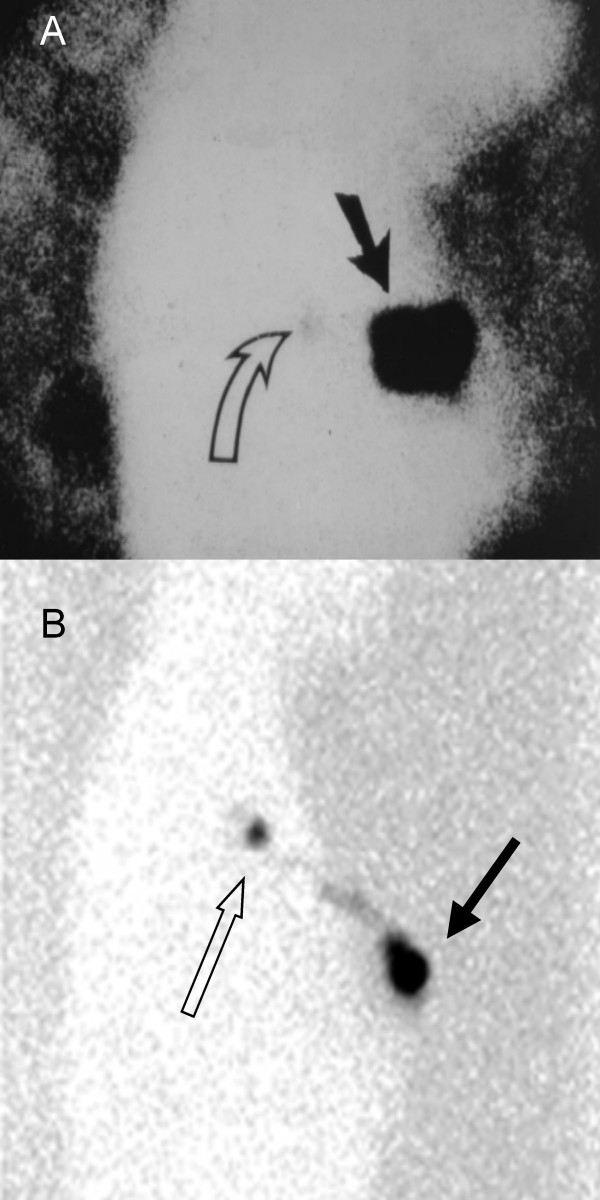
(A*) TOP: Labeled as a "typical" lymphoscintigraphy finding in an article disputing the value of lymphoscintigraphy, the lateral view depicts the poor quality of injection and imaging technique [37]. The injection site is represented by the solid arrow, the faint, barely visible SN by the open arrow. (B) BOTTOM: Right lateral view of typical/average result from optimized injection and imaging protocol showing injection sites (solid arrow) and bright sentinel node (open arrow) as well as lymphatic channel leading to sentinel node [38]. In many cases, even much brighter nodes than depicted in B are found. Reprinted from Am J Surg. 177, Burak WE Jr, Routine preoperative lymphoscintigraphy is not necessary prior to sentinel node biopsy for breast cancer, 445–449., 1999, with permission from Elsevier Ltd.; Excerpta Medica Inc *. [37].
